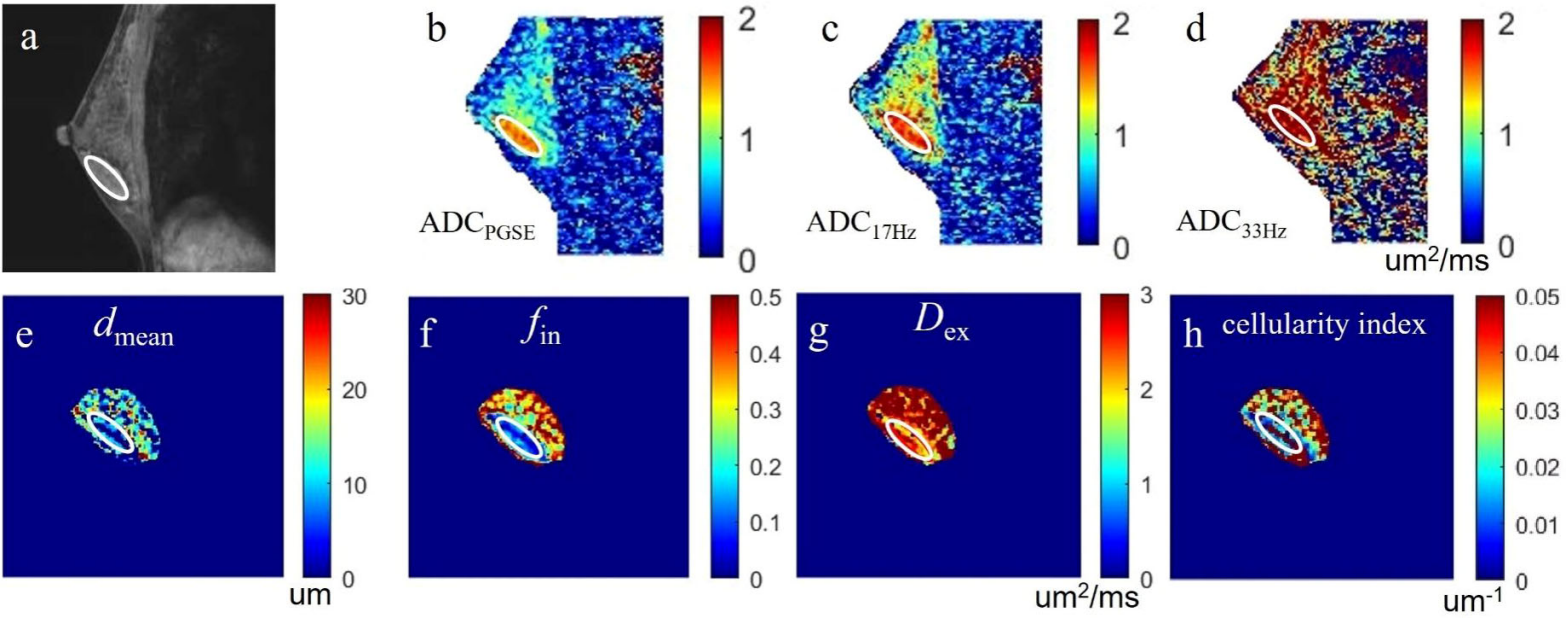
a case of fibroadenoma in the right breast: (A) the sagittal DCE-MRI image as reference; (B-D) the sagittal ADC_PGSE,_ ADC_17Hz_, and ADC_33Hz_ images with values for the lesion of 1.382, 1.589 and 2.523 um^2^/ms, respectively; (E-H) the *d*
